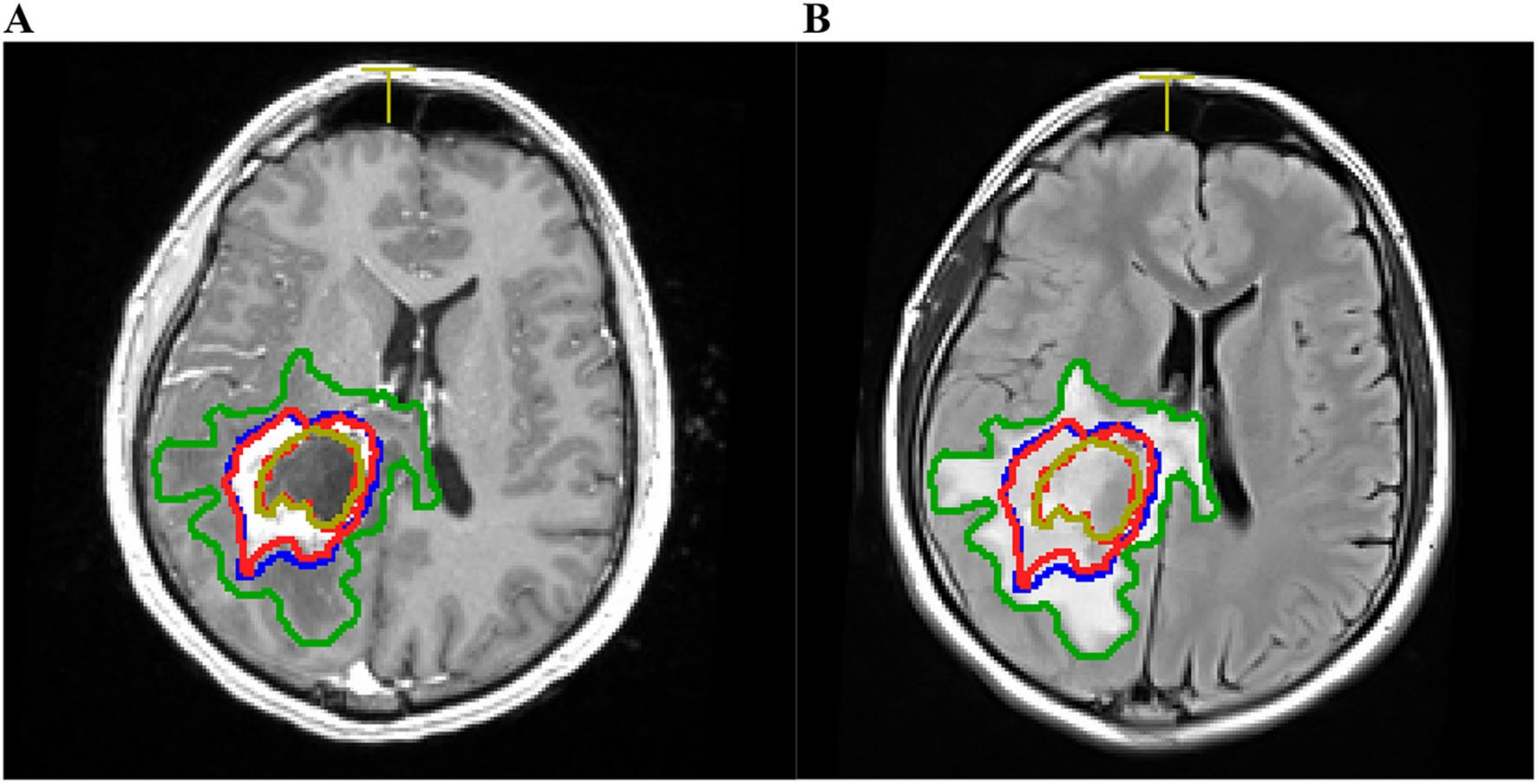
Manual segmentation of the glioblastoma compartments on T1-weighted contrast-enhanced MRI and T2-Flair MRI sequences. GTV (gross target volume) in blue, CE (contrast-enhanced region) in red, NEC (necrosis areas) in yellow, FLAIR in green in axial slice of (A) T1-weighetd contrast-enhanced MRI sequence, (B) T2-Flair MRI sequence.
